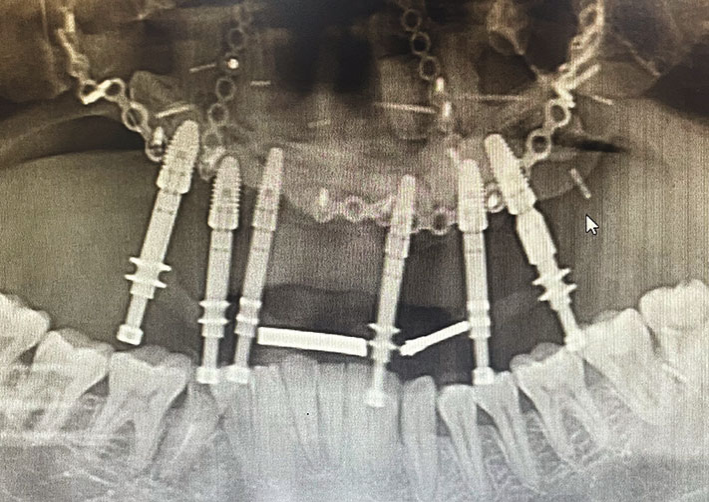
Orthopantomography showing marked bone loss.
